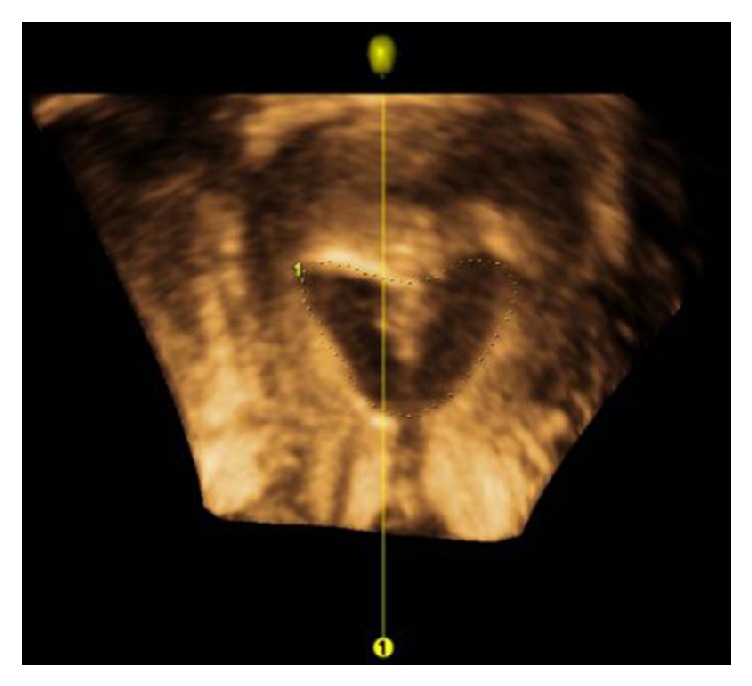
Ultrasound images of the intrauterine cavity in the ISB.
